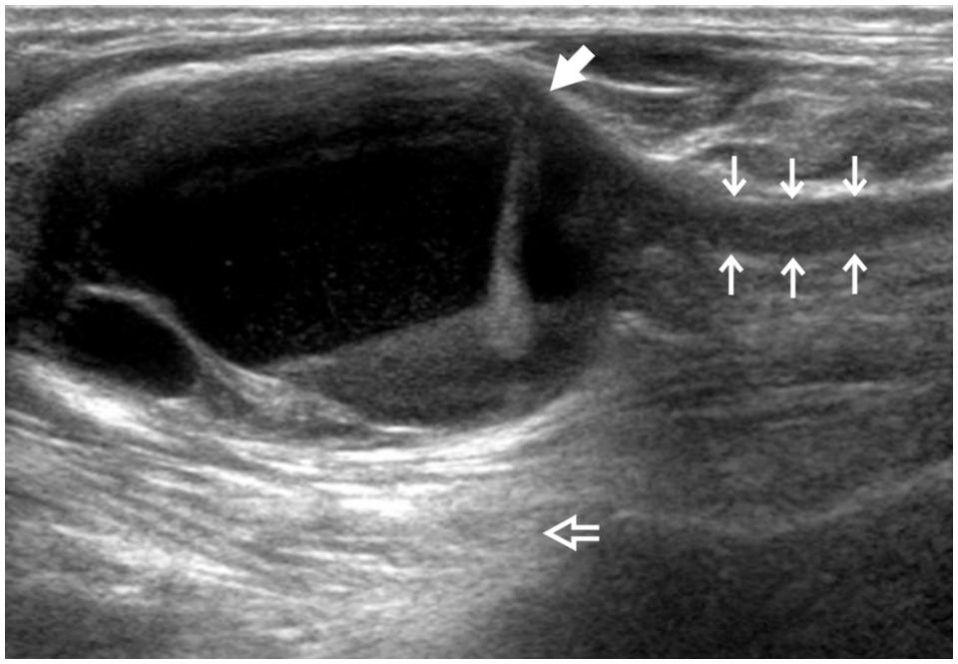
Longitudinal ultrasound showing a medium-sized nerve sheath tumour arising from the C5 root of the brachial plexus, which is in continuity with the tumour. The tumour (thick arrow) shows severe cystic degeneration with moderate posterior enhancement (open arrow).
